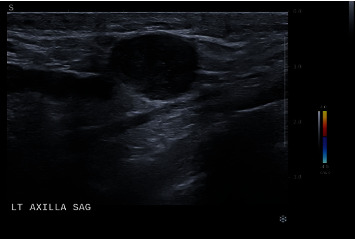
Ultrasound analysis of the patient's left breast which revealed a BI-RADS category 4 irregular, indistinct, and hypoechoic mass measuring 2.0 × 1.5 × 1.2 cm at the 1 : 00 position, 4 cm from the nipple with the left breast.
